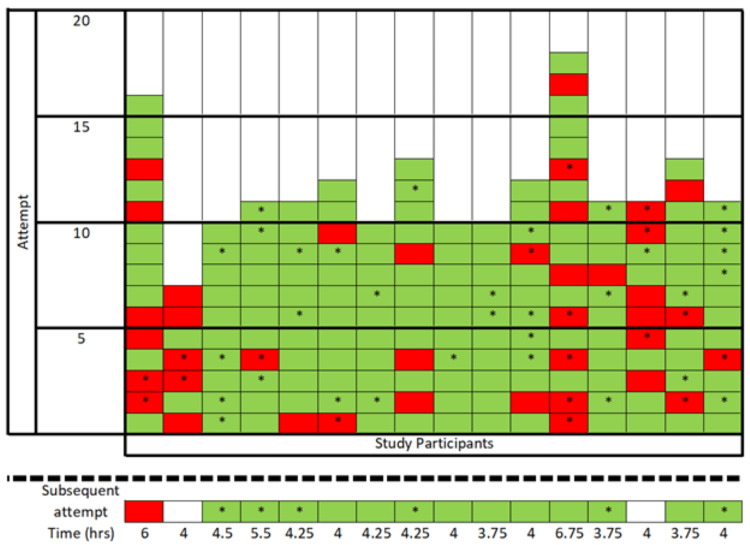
Individual performance of each USGPIV attempt during direct observation and subsequent USGPIV attempt after 10 successful insertions The total observer time is listed for each subject. * = DIVA; green = successful USGPIV; red = unsuccessful USGPIV; white = no attempt DIVA, difficult intravenous access; USGPIV, ultrasound-guided peripheral intravenous
